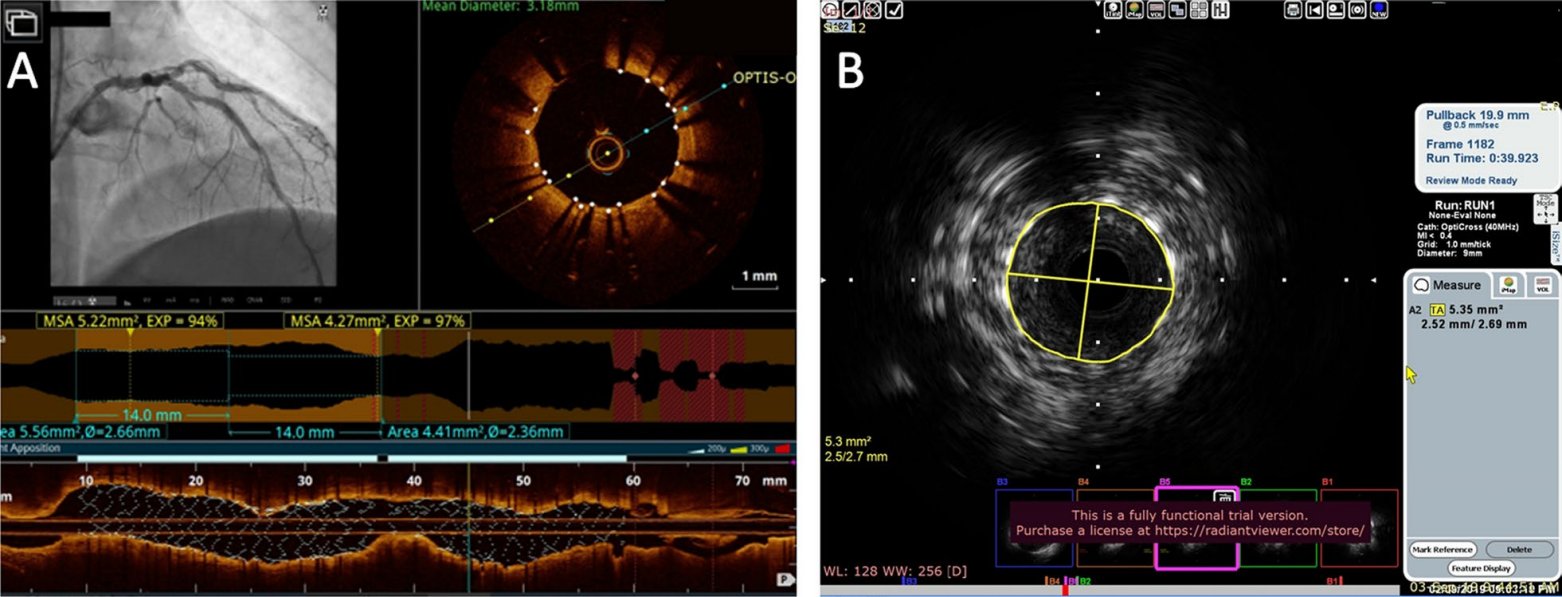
A OCT image in a patient with deployed stent in the LAD showing well apposed stent struts with MSA of 5.2 mm and expansion of 94% B IVUS image showing adequate stent apposition and expansion and MSA of 5.3 mm. *OCT* optical coherence tomography, *IVUS* intravascular ultrasound, *LAD* left anterior descending coronary, *MSA* minimal stent area
